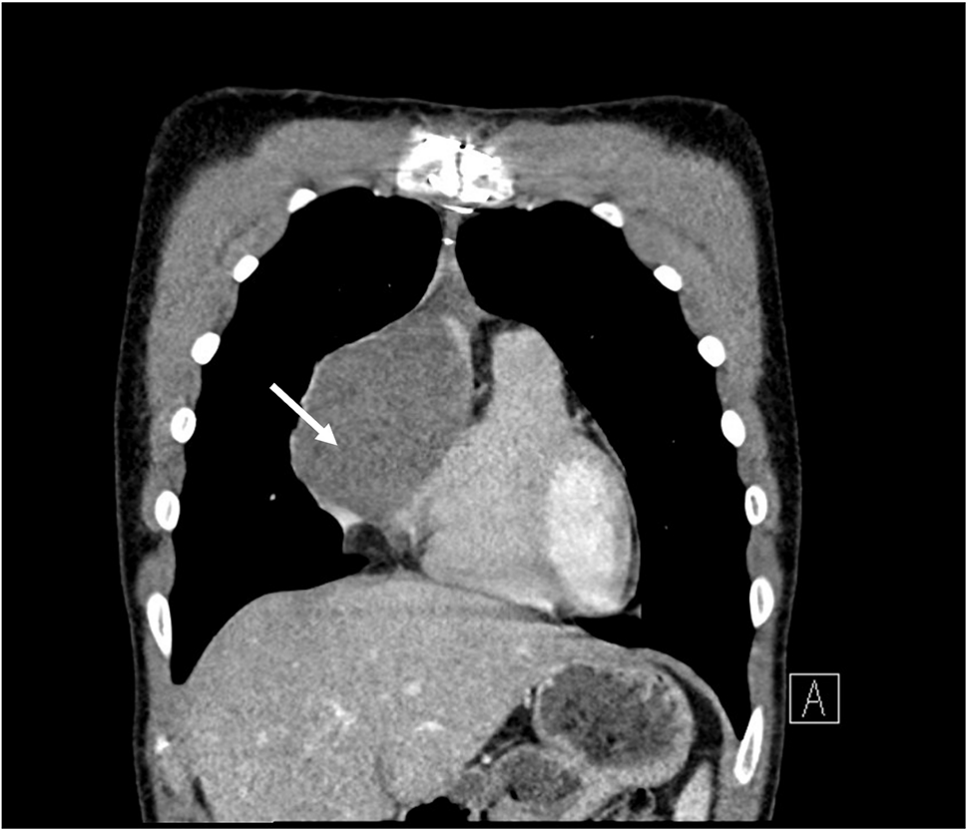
CT coronal slice confirming increase in size of the cardiac angiosarcoma (arrow)
